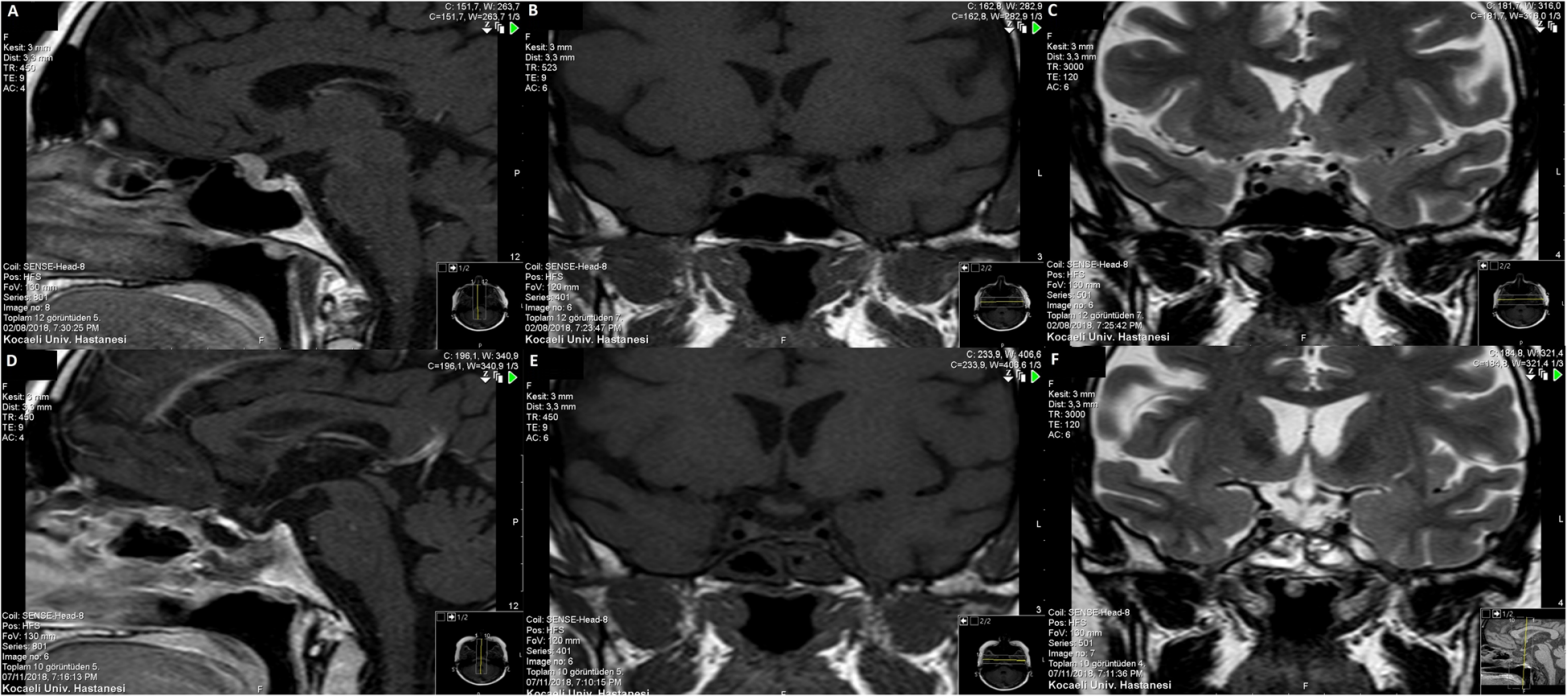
Preoperative and postoperative gadolinium-enhanced magnetic resonance images of the sellar region. a–c Before transsphenoidal surgery, T1-weighted with contrast sagittal, T1-weighted without contrast coronal, T2-weighted without contrast coronal views, respectively, suprasellar/sellar lesion, in close relation to optic chiasm. d–f After transsphenoidal surgery, T1-weighted with contrast sagittal, T1-weighted without contrast coronal, T2-weighted without contrast coronal views, respectively, tumor resection
